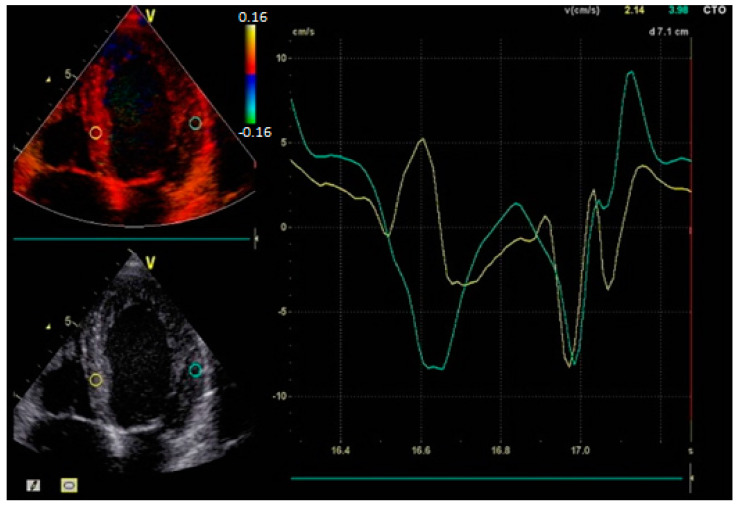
Echocardiographic assessment of a patient with left bundle branch block. Tissue Doppler imaging highlighting interventricular dyssynchrony.
